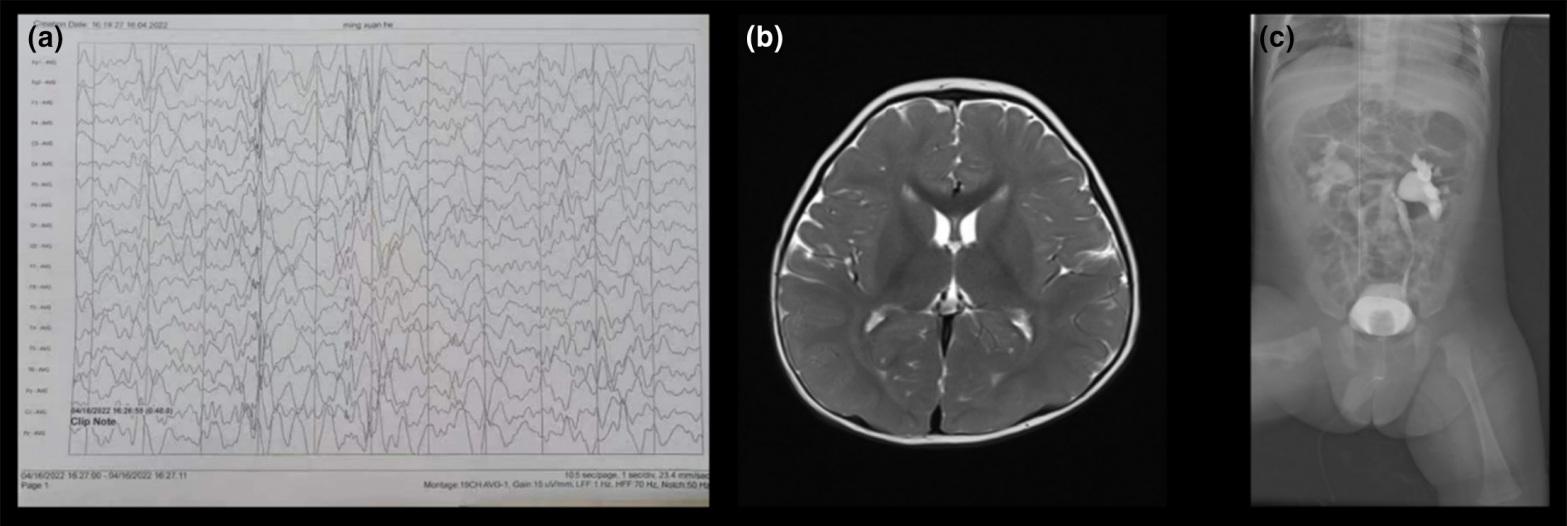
Clinical information of the patient. (a) Electroencephalogram showing an abundance of sharp low‐to‐high amplitude waves and 2.5–4 Hz spike‐and‐slow waves in the frontal and central regions during sleep. (b) Magnetic resonance image of the head showing no abnormalities. (c) Cystourethrography indicating the presence of the vesicoureteral reflux.
